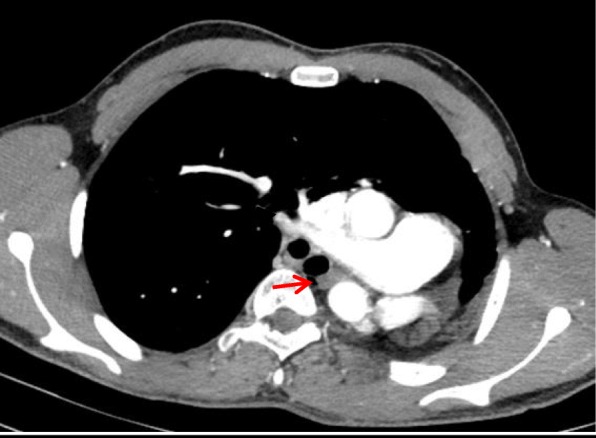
Axial CT image of eighteen years old boy in mediastinal window shows absent left lung with rudimentary left bronchus (red arrow) with left posterior position of cardiac and great vessels and right lung significant extension towards the left hemithorax
